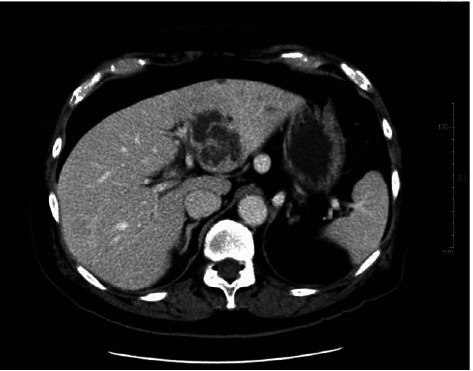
Contrast-enhanced computed tomography on arrival which shows multilocular abscess cavities with a mild enhancement.
